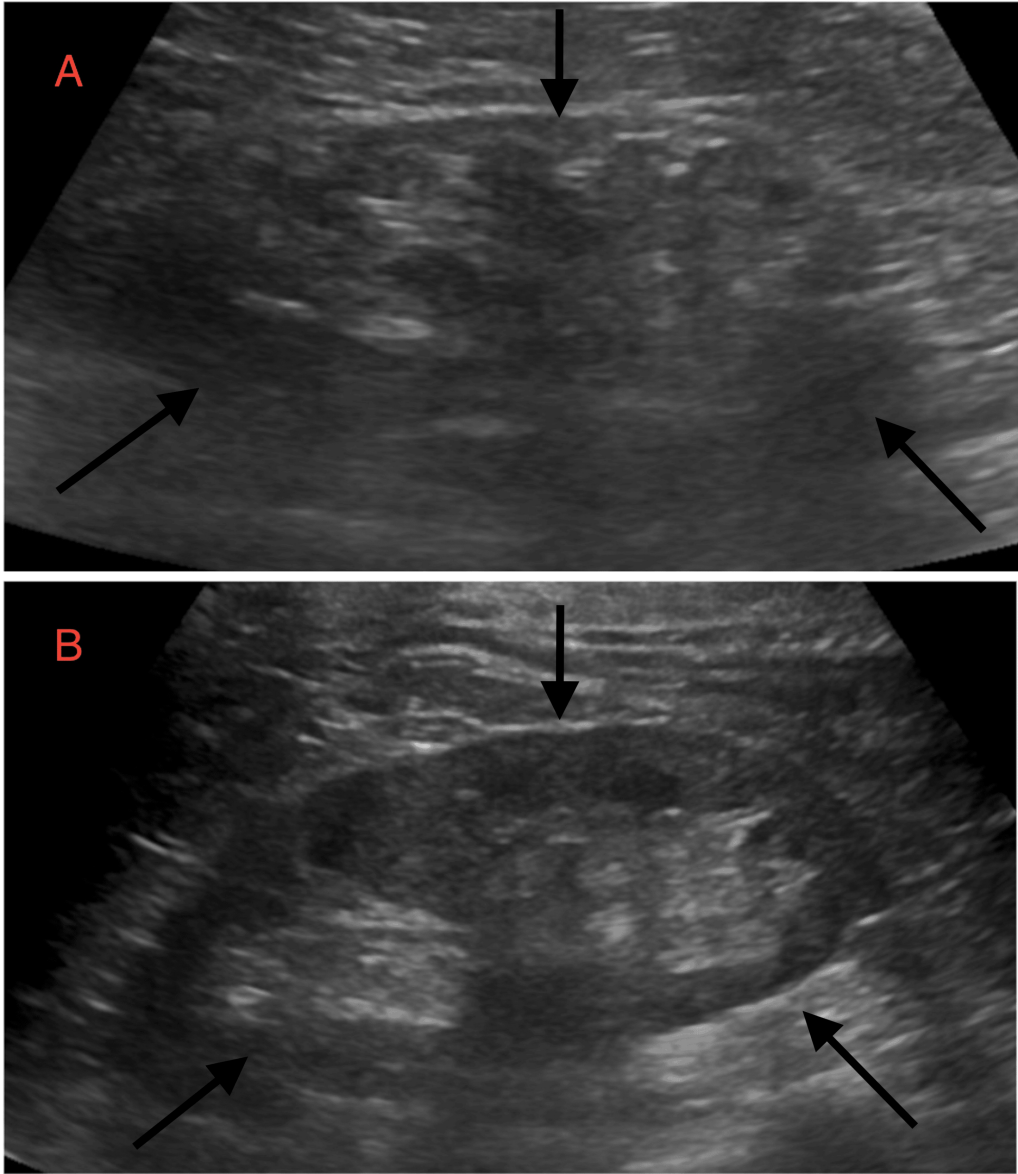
Renal ultrasound revealed a left kidney measuring 9.1 × 3.6 × 4.3 cm (A) and a right kidney measuring 10.7 × 1.0 × 1.9 cm (B). Although sonographic findings were unremarkable for RAS, the left kidney appeared mildly atrophic. Arrows indicate the dimensions and surrounding anatomical context of each kidney RAS: renal artery stenosis
